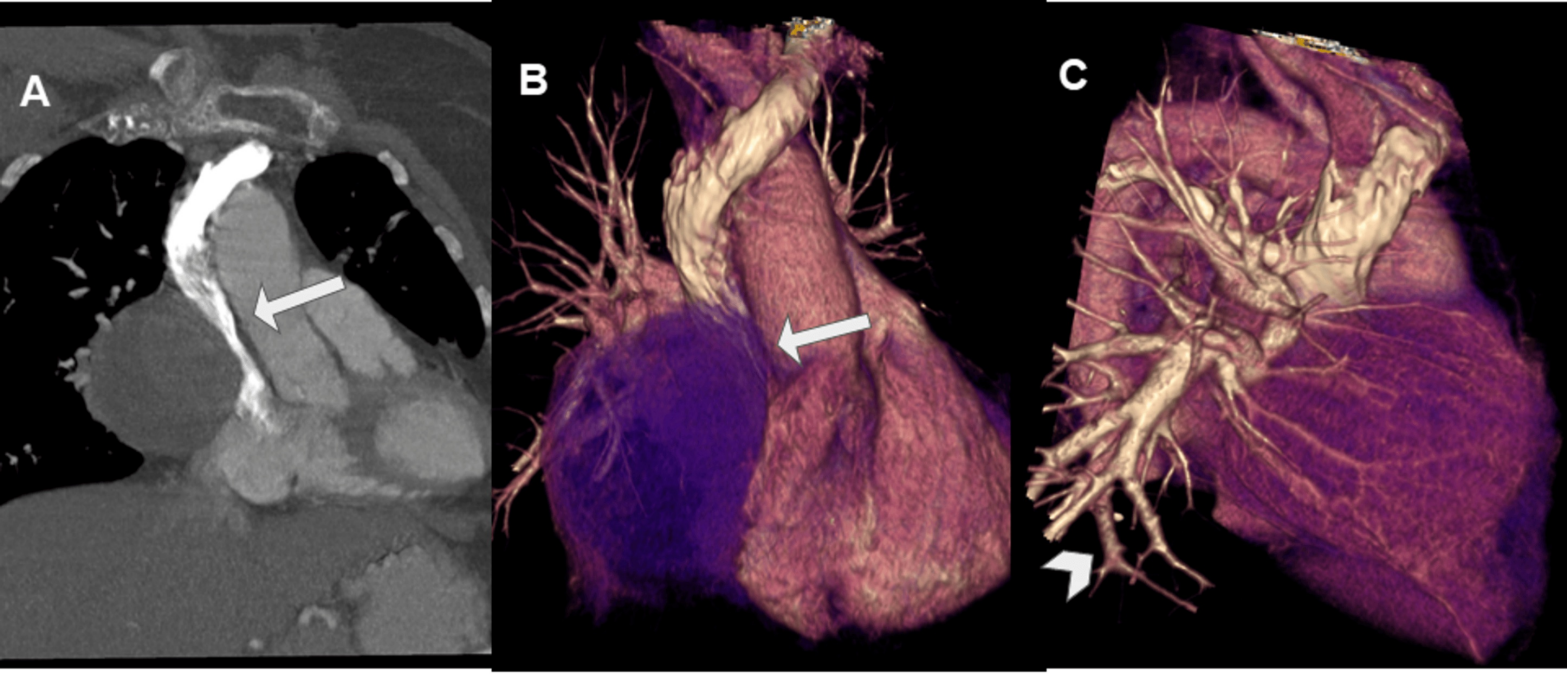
Multiplanar (A, coronal view) and 3D (B and C) reconstructions of the contrast-enhanced CT. These post-processed images were useful for eventual surgical planning, confirming the extrinsic compression of the SVC (arrow in A and B) and showing abundant feeding vessels of the tumor originated from the pulmonary artery (arrowhead in C). CT: computed tomography; SVC: superior vena cava.
